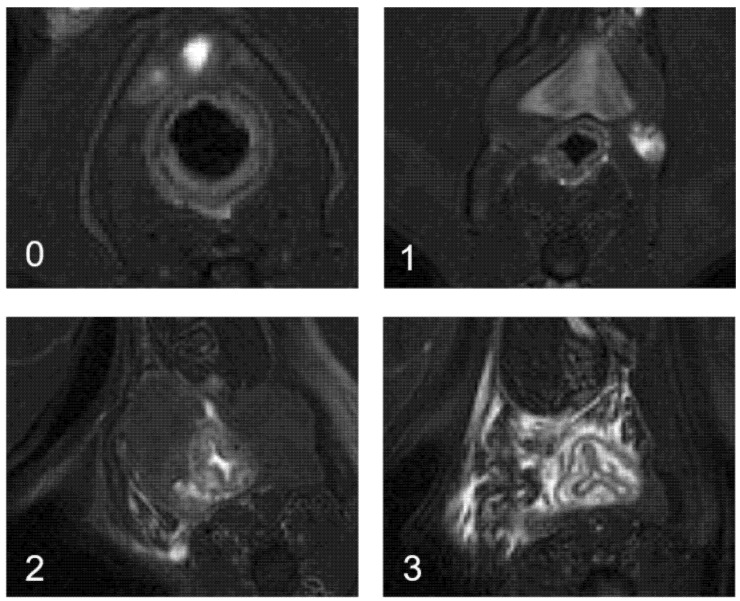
Global proctitis scores (GPS) from T2-weighted morphological images by MRI. 0: no proctitis, no inflammation in the rectal wall, nonmodified signal; 1: mild proctitis, hypersignal in the rectal wall; 2: moderate proctitis, hypersignal in the rectal wall + peri-digestive oedema; and 3: severe proctitis, hypersignal in the rectal wall + peri-digestive oedema + submucosal oedema with cocarde appearance.
